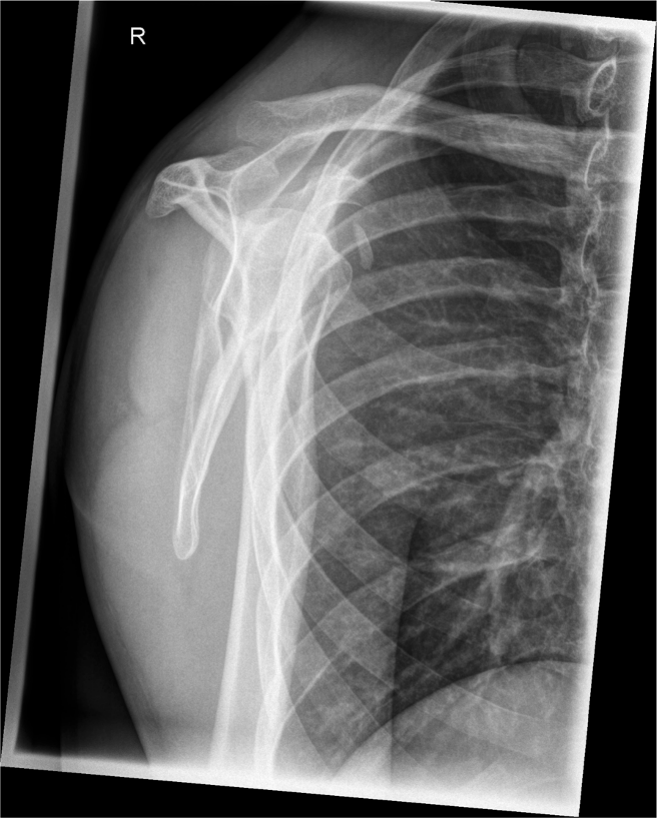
Preoperative X-rays in lateral projection.
